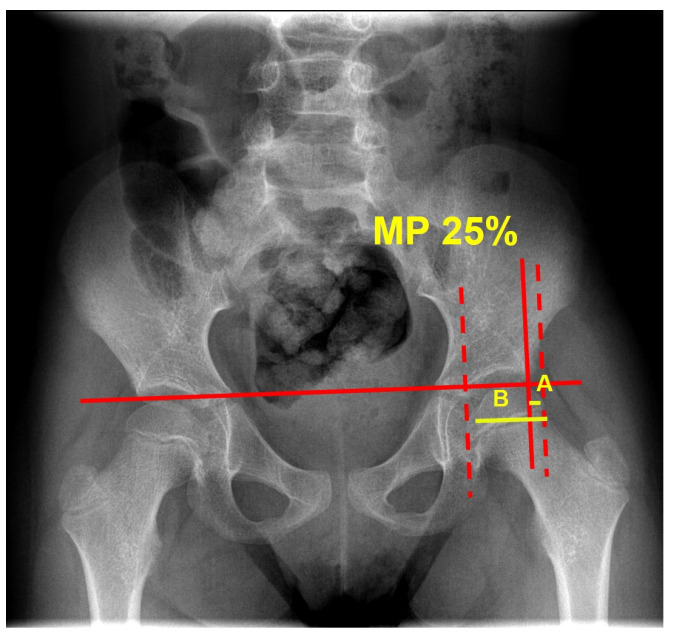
Example of an anterior–posterior radiograph of the pelvis with calculation of the migration percentage (MP): MP = A/B × 100. A represents the portion of ossified femoral head laying lateral to Perkin’s line (vertical line drawn through the lateral acetabular margin and perpendicular to Hilgenreiner’s line, which passes through the superior aspect of the triradiate cartilage). B represents the whole ossified femoral head.
